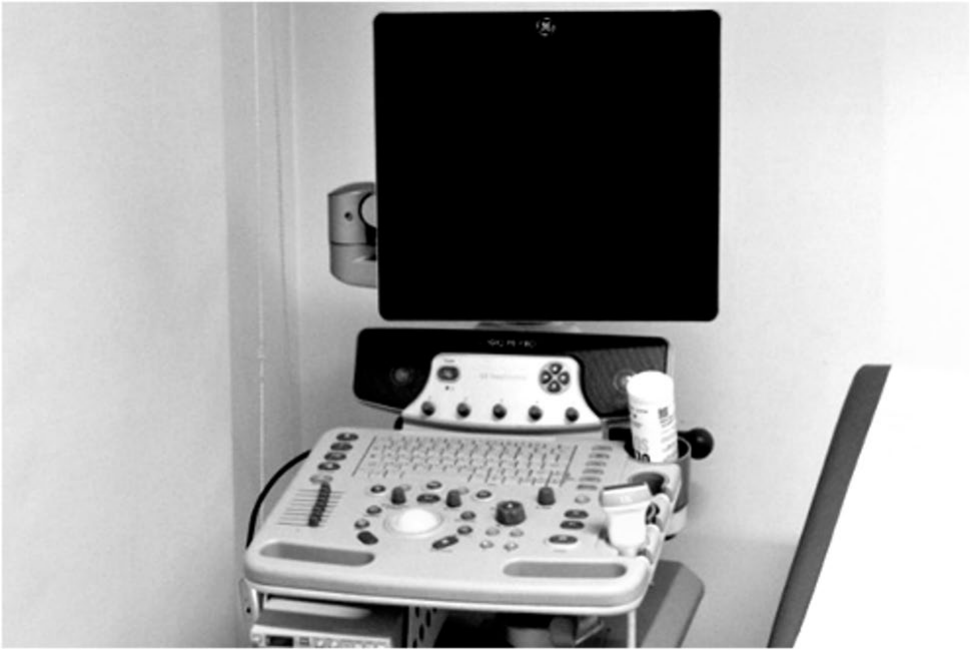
General electric LOGIQ P6 ultrasound system
